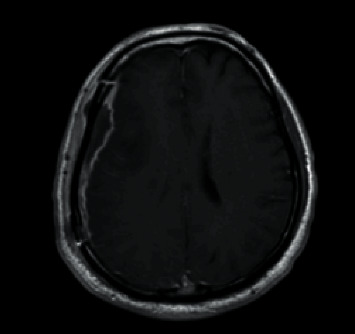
Axial postcontrast T1-weighted MRI brain showing a subdural collection along the right convexity measuring up to 0.9 cm in thickness.
